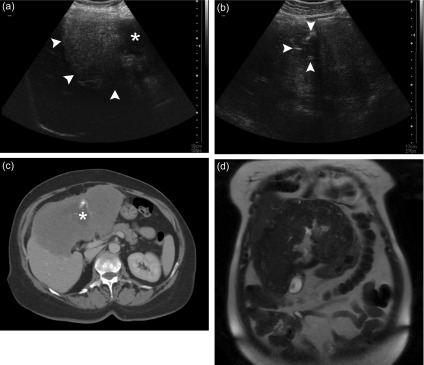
A 43-year-old female patient presented with abdominal pain and malaise. (a) Abdominal ultrasound examination reveals a hyperechoic, heterogeneous solid mass lesion that fills part of the right lobe and the entirety of the left lobe of the liver. Its borders can be clearly discerned from the adjacent normal hepatic parenchyma (*arrowheads*). The necrotic cavity in the central zone of the lesion appears as a hypoechoic area with irregular contours (*asterisk*). (b) Calcifications around the central necrotic zone can be discerned as hyperechogenic foci (*arrowheads*). (c) An axial contrast-enhanced CT image demonstrates the hypovascular solid mass lesion more clearly. The necrotic cavity in the left lobe (*asterisk*) and the tiny calcifications around it are visible. Additionally, note that the lesion causes a retraction in hepatic contours. (d) A coronal T2W MRI image shows a heterogeneous mass lesion that is mildly hypointense relative to liver parenchyma. While the necrotic cavity in the center of the lesion appears of high signal intensity, the calcifications are not easily discernible.
